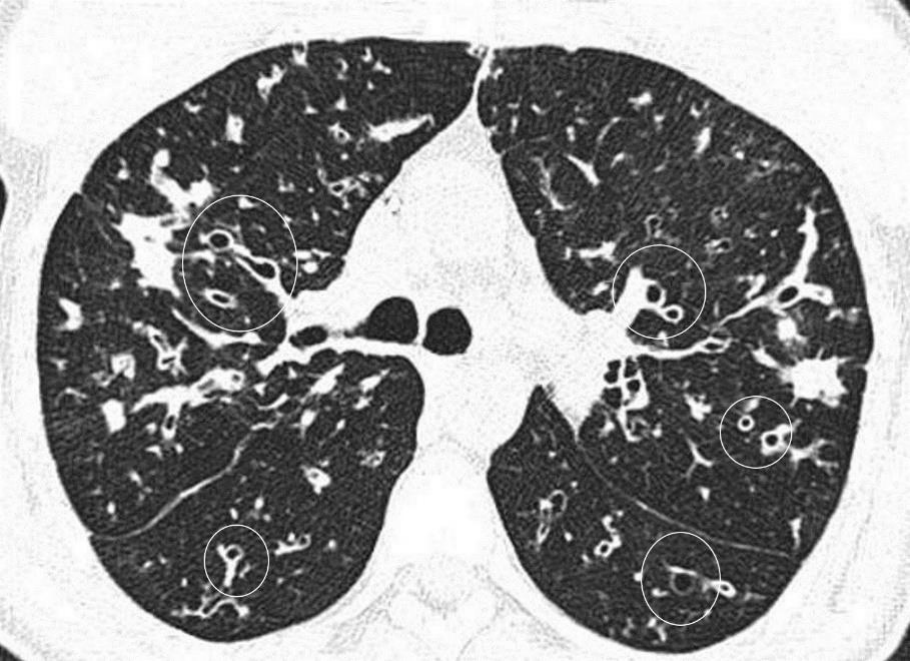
An axial CT image (lung window) of the chest of a 24 years old female with history of cystic fibrosis shows multiple irregular opacities and cystic changes in both the lungs. Cystic changes (circled) are suggestive of bronchiectasis. Note the thickened wall of dilated bronchi. Right oblique fissure is also mildly thickened
